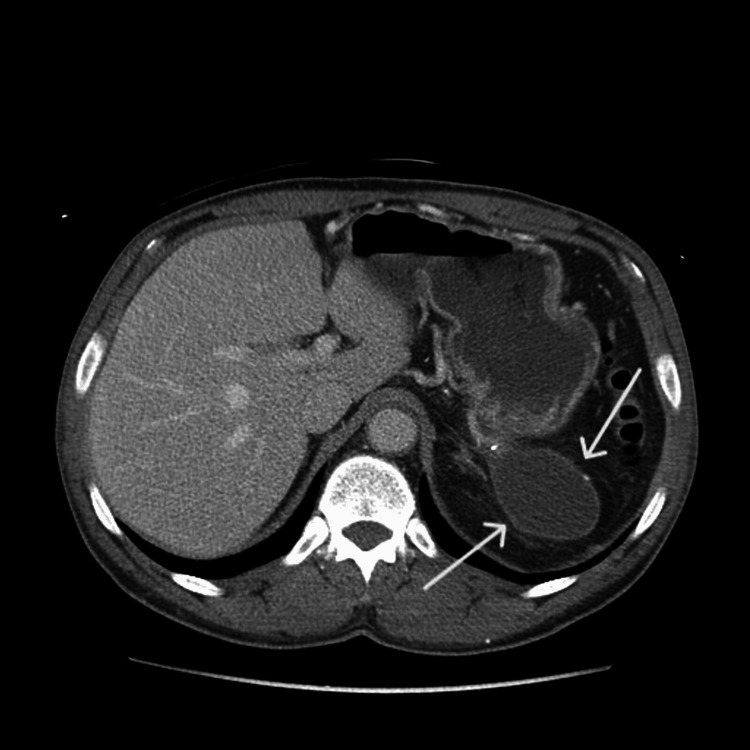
Abdominal CT with intravenous contrast showing a pseudocyst.
